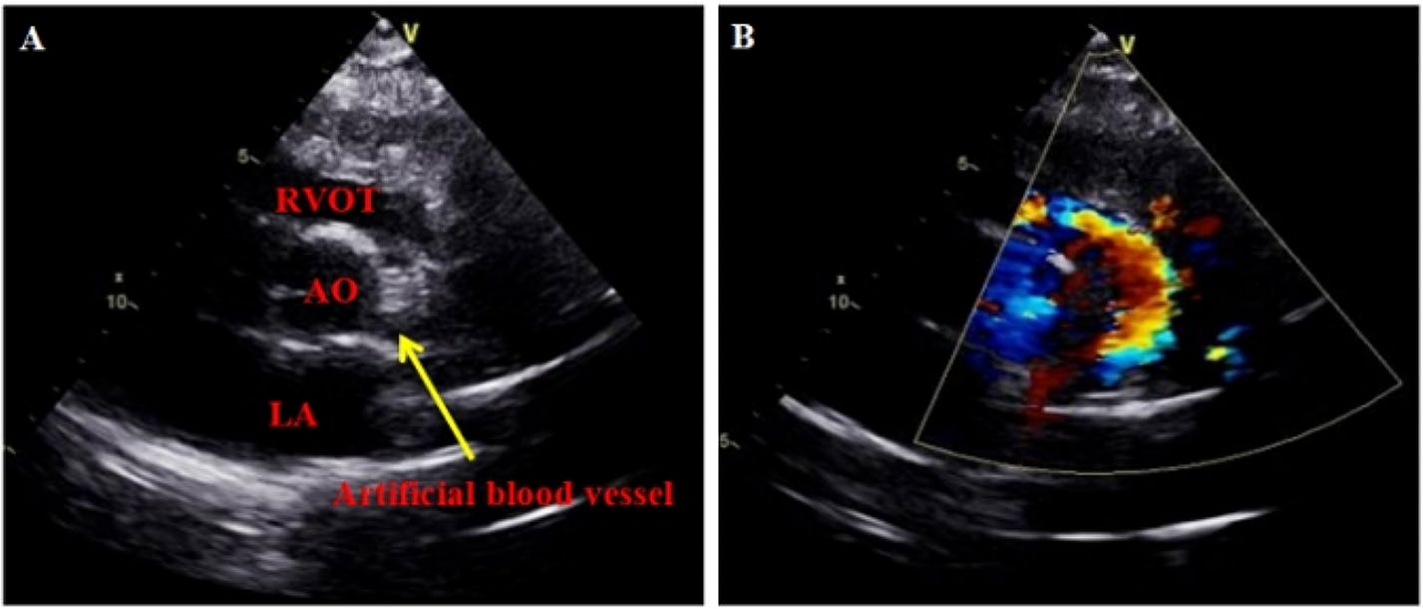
Postoperative color doppler echocardiography. A A 7 mm wide tubular echoless area - artificial blood vessel (yellow arrow) could be seen at about 4 o ‘clock on the short axis section of the great artery. B Color blood flow Doppler imaging indicated that star point blood flow signals could be seen in the myocardium of ventricular septum and left ventricular wall. AO: aorta, LA: left atrium, RVOT: right ventricular outflow tract, Artificial blood vessel: artificial blood vessel
